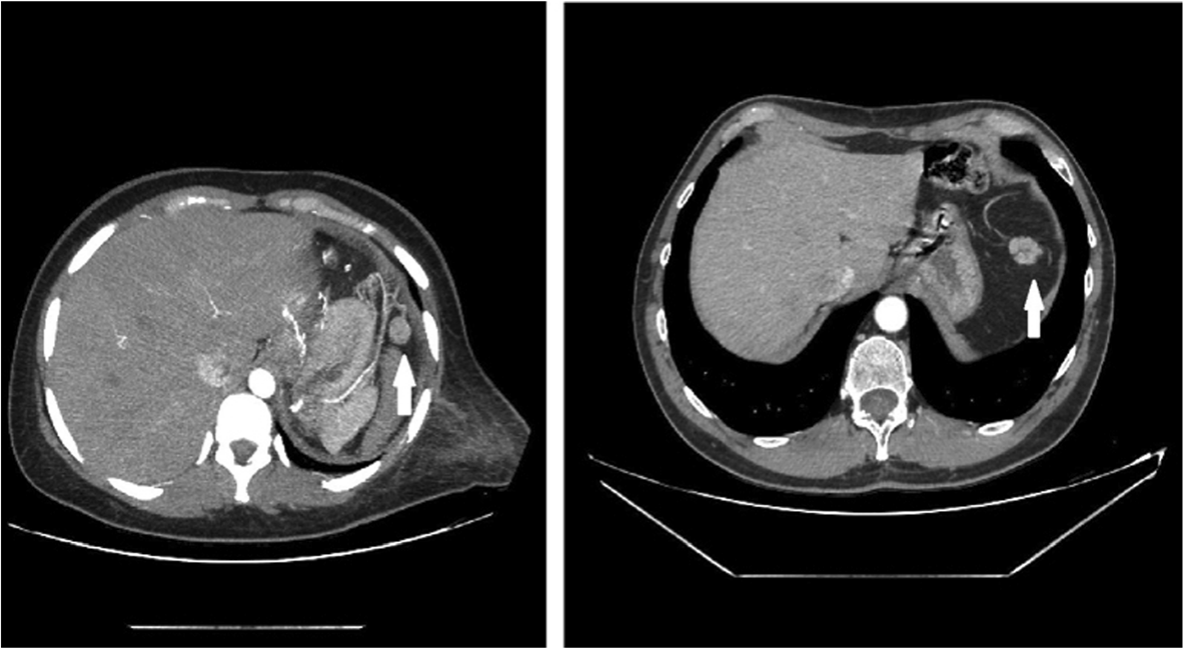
CT-scan images of two of the patients who underwent spleen autotransplantation. White arrows show the position of the new growth of the transplanted splenic parenchyma
